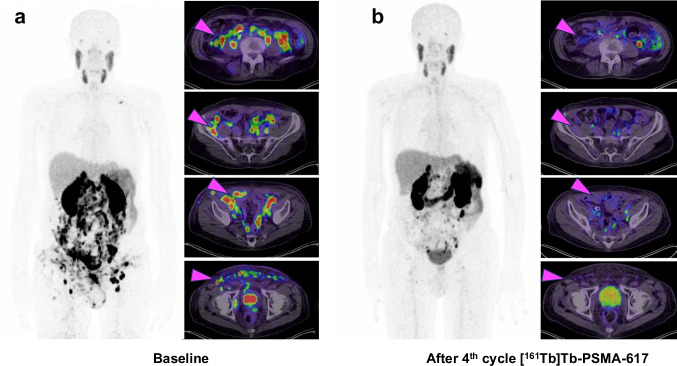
A 72-year-old mCRPC patient with diffuse peritoneal, multiple lymph node, and soft tissue metastases (exemplary magenta arrows) received PSMA RLT with [^161^Tb]Tb-PSMA-617. a) depicts a [^68^Ga]Ga-PSMA-11 PET/CT scan pre PSMA RLT and b) depicts a [^68^Ga]Ga-PSMA-11 PET/CT scan after four cycles of [^161^Tb]Tb-PSMA-617 RLT with regression of metastases
